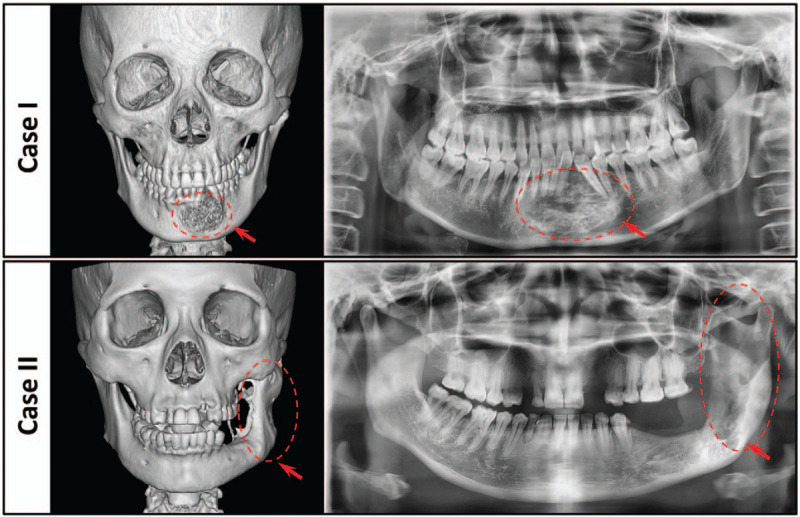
Preoperative CT images and panoramic radiographs of the patients. Case I: trans-midline bilateral mandibular defect (ameloblastoma located in the middle of the mandible). Case II: hemi-mandibular defect (ameloblastoma located in the left mandibular angle and ramus region). Red arrows and dotted lines indicate tumor areas. CT = computed tomography.
